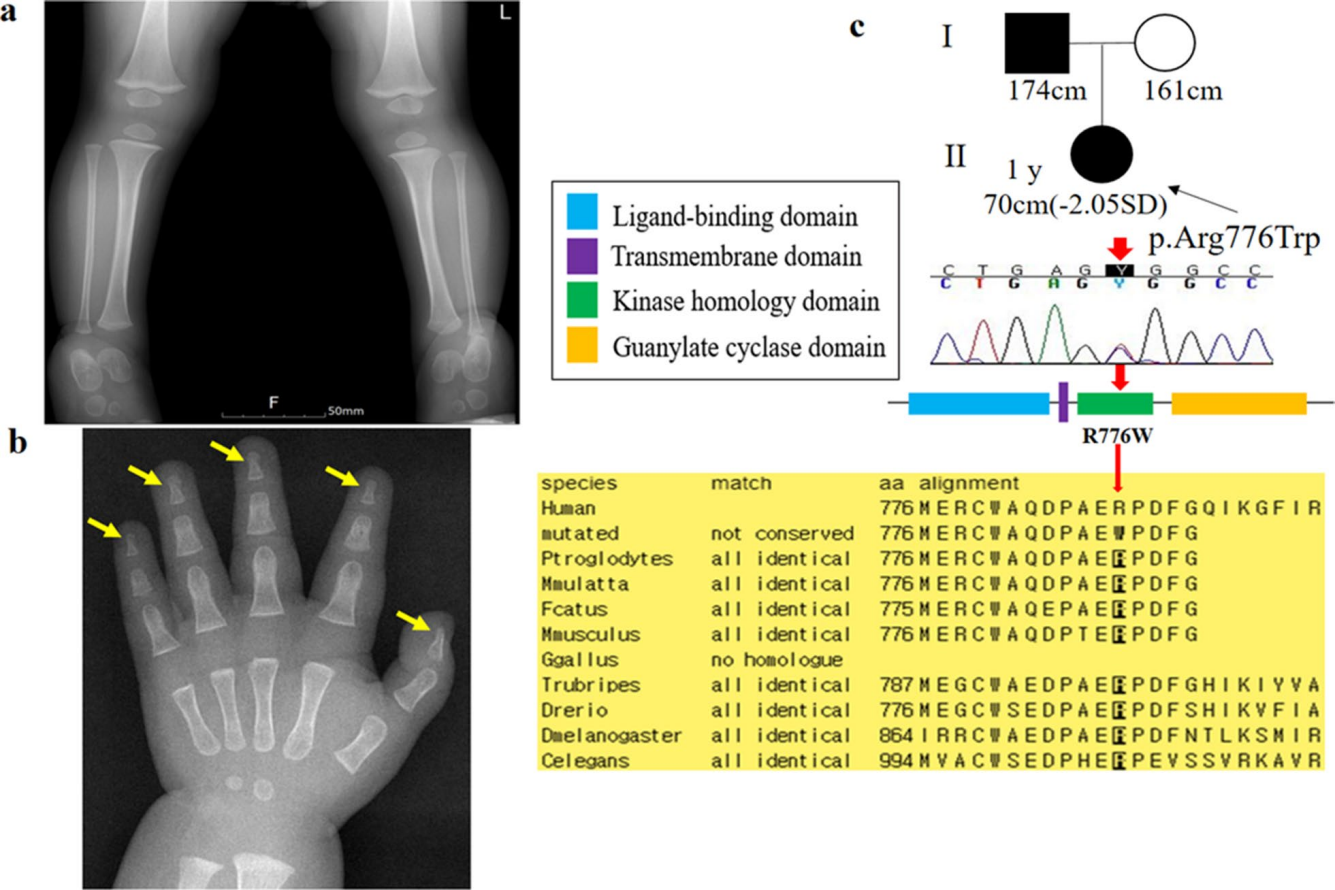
Radiographic image and molecular analysis of patient K9 with acromesomelic dysplasia Maroteaux-type. a Lower extremities X-rays demonstrated a short tibia and fibula, with no missing or fused bones; b phalanges of the hands were short and broad with cone-shaped epiphyses, especially in the 4th proximal phalange marked by yellow arrows; c Sanger sequencing confirmed a missense mutation, c.2326C>T (p.A776W) (NM_003995.3), in the kinase homology domain of the *NPR2* gene in the proband and her father. The A776 residue is conserved among species (dark square box). Sequences were aligned using blastp [https://blast.ncbi.nlm.nih.gov/]
